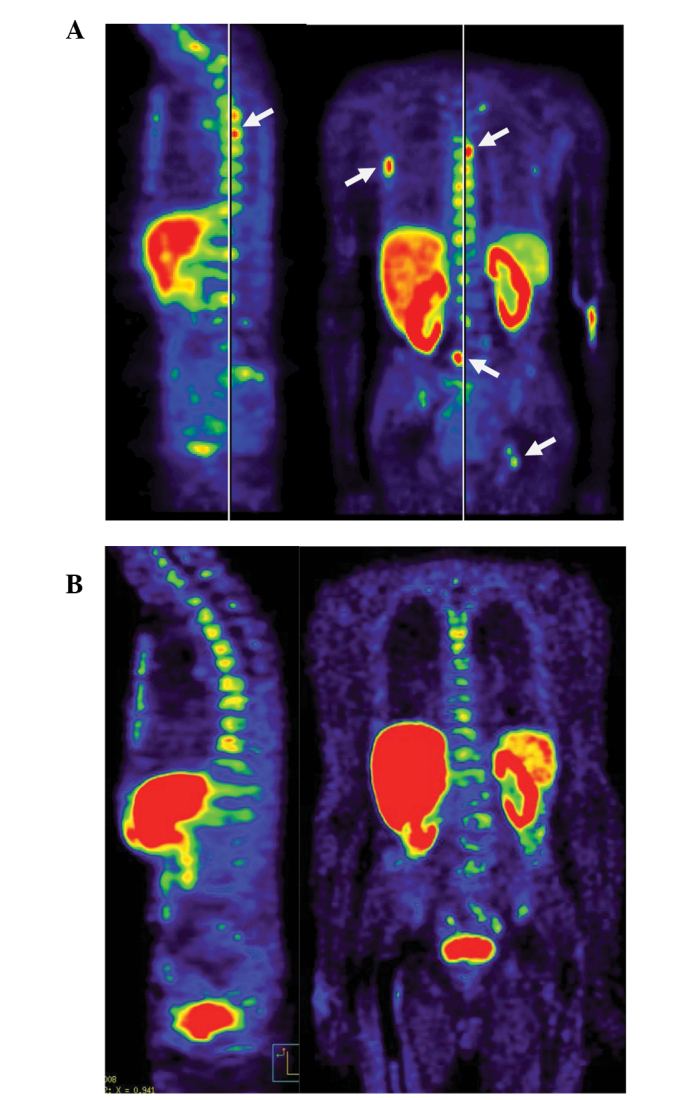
^18^F-choline-positron-emission-tomography/computed tomography scans. (A) From September 2007, prior to immunotherapy. Multiple focal tracer enhancements in the spine, 5th right rib and pelvis. (B) From March 2008, following immunotherapy. Distinct regression of skeletal metastases, no more focal enhancement observable. Mild diffuse tracer enhancement in thoracic spine, representing low remaining metabolic activity.
